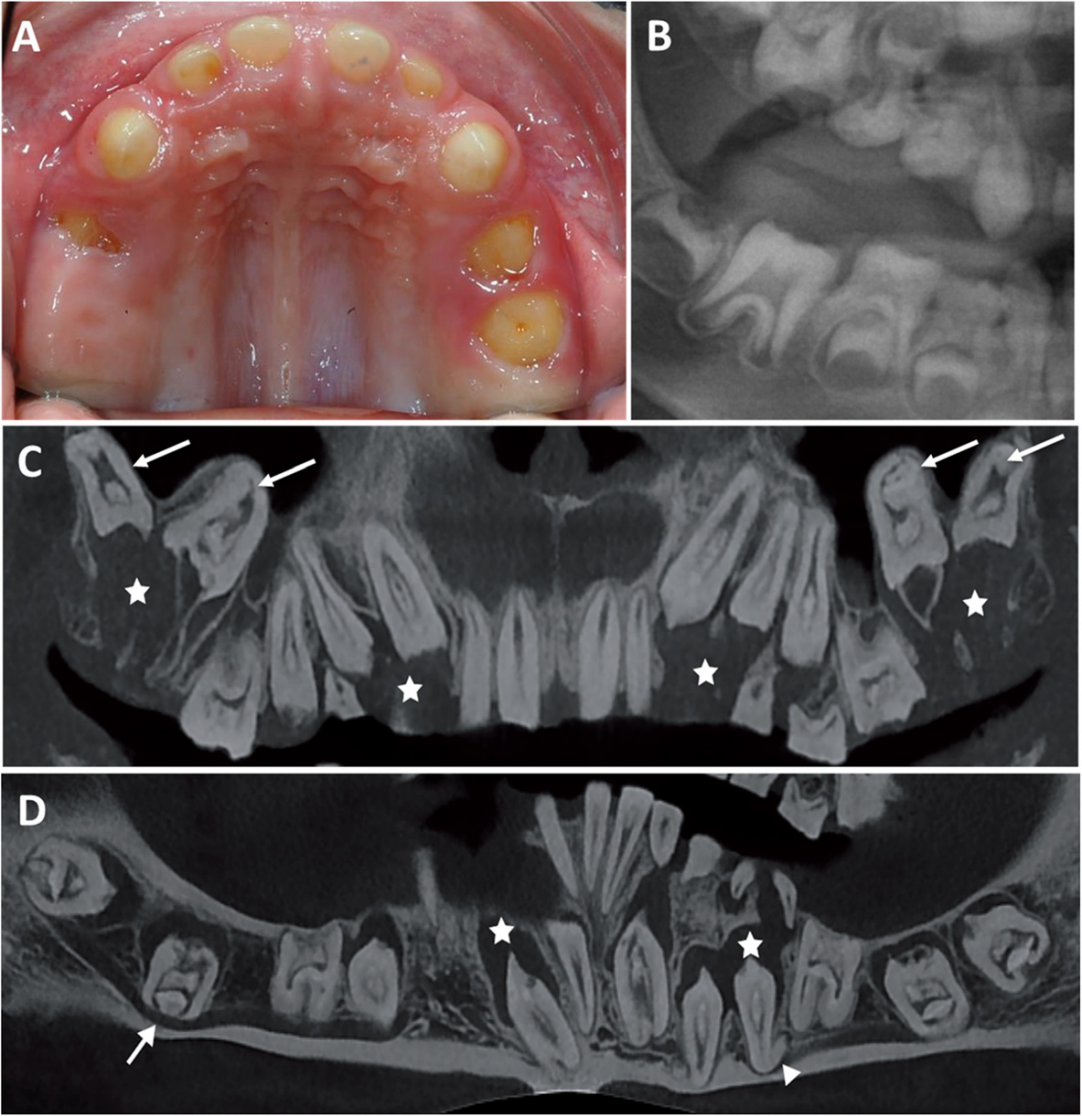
Eruption anomalies of ERS patients. (A, B) Oral and radiographic view of no erupted or partial erupted temporary molars in a 5 years old patient. (C, D) Cone beam radiograph of maxillary and mandible arch showing hyperplasia of dental follicle (star), dilacered root (triangle), inverted erupting pathway : maxillary molar is seen in the sinus and mandibular molar crossed cortical bone of mandible (arrow).
